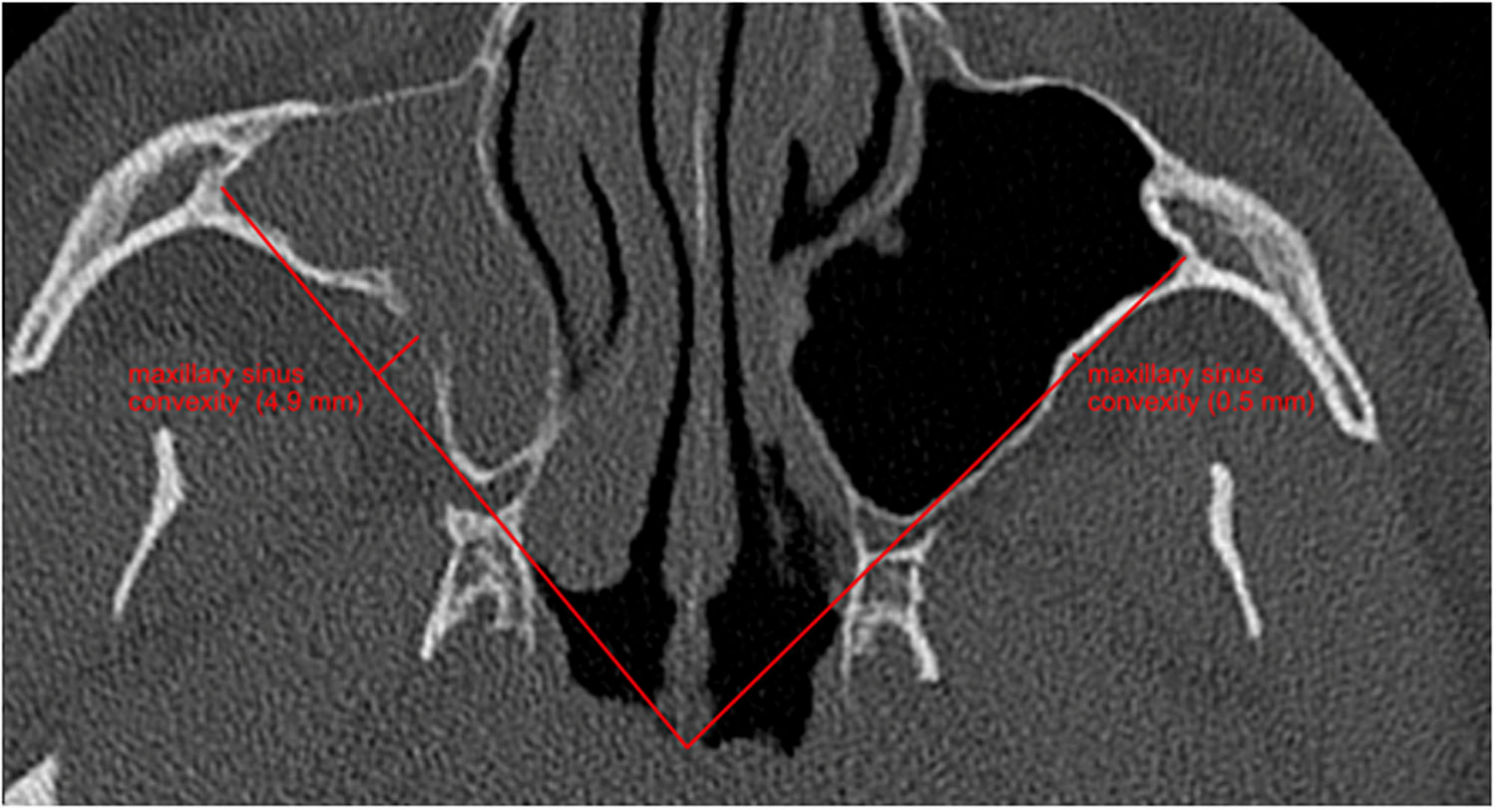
Measurement of the posterior wall convexity of the maxillary sinus in the axial CT scan with a left healthy sinus and an SSS‐affected sinus on the right side. CT, computed tomography; SSS, silent sinus syndrome.
